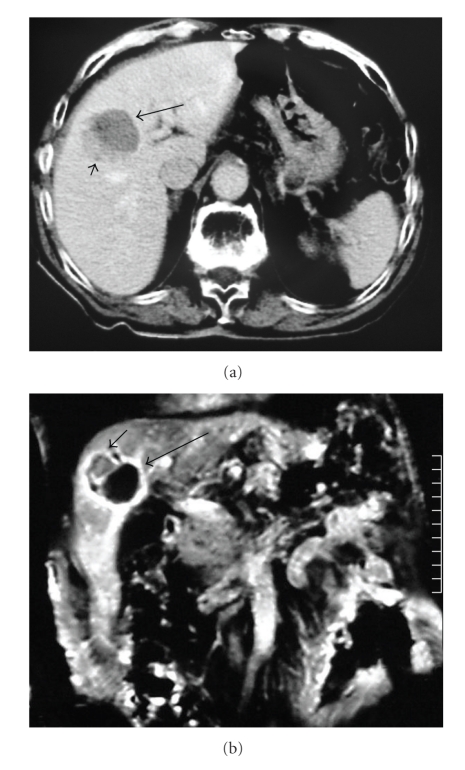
(a) After 18 months, a new CT scan depicted a local tumor progression at the ablation site. Note the unenhanced area of the lesion corresponding to the ablated site (long arrow) and the local tumor progression seen as an adjacent hypodense area (short arrow). (b) Similar to findings on the MRI scan, the ablated area shows no signal (long arrow), whereas the local tumor progression appears as a low signal area (short arrow).
